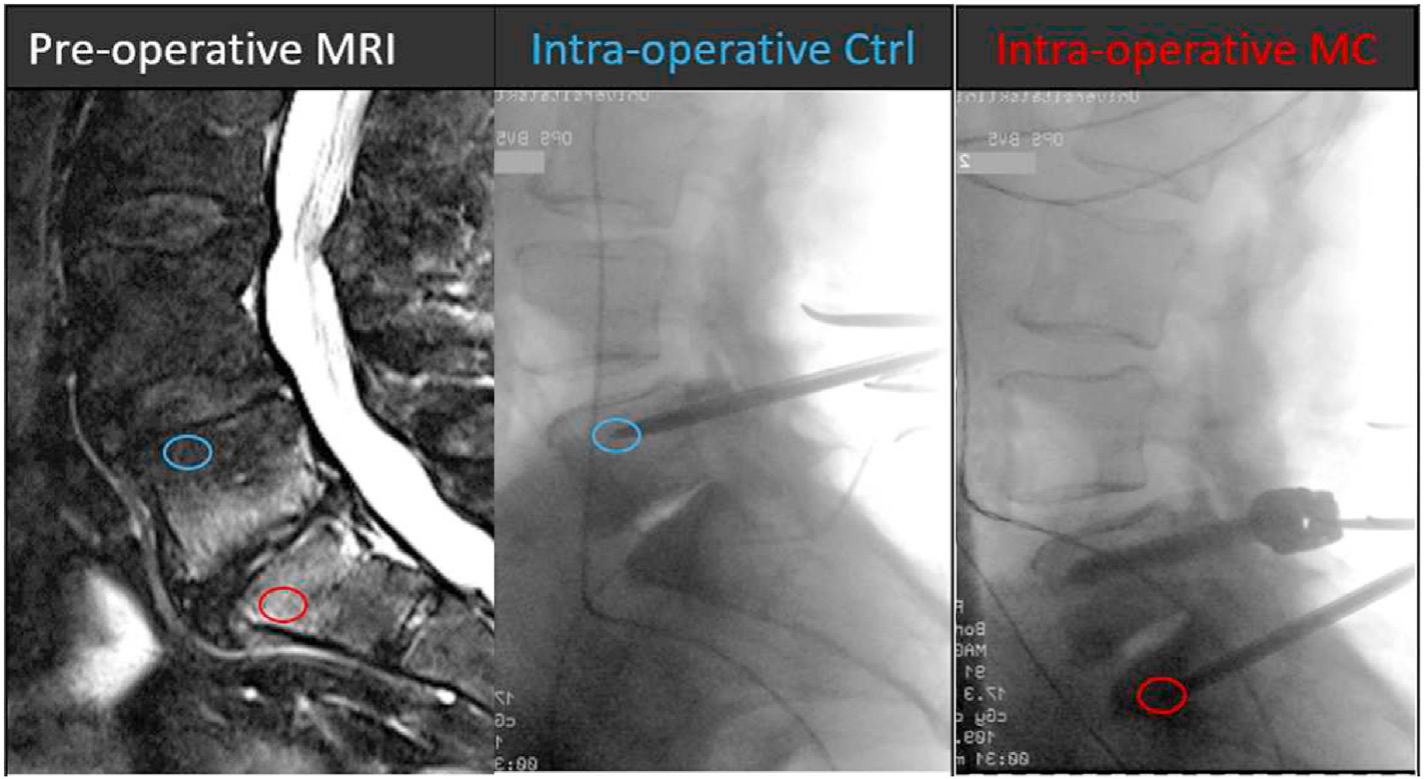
Intra-operative sampling of vertebral bone marrow biopsies. Patients with lumbar MC were identified pre-operatively on MRI (left). Biopsies were taken intra-operatively with Jamishidi needles (middle and right). Intra-operative X-ray was used to confirm proper needle position. A control (blue circle) and a MC (red circle) biopsy were taken.
